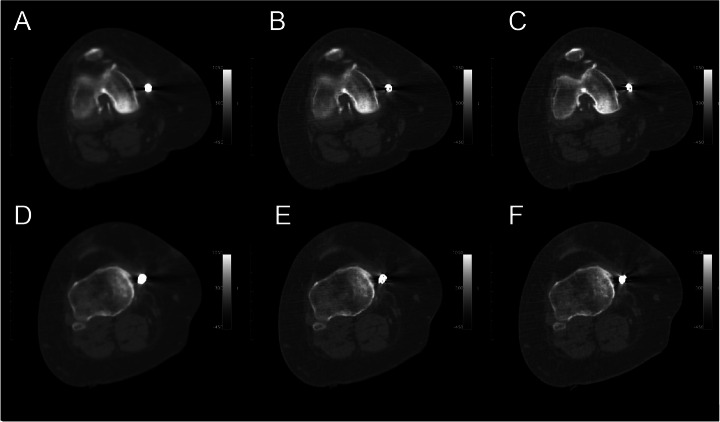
Low-dose CT scan of a female patient (48 year) with an Atlas® Knee System in the right joint. Multiple reconstructions from the same CT detector raw data (120 kV, 55 mA, *t*_rot_ = 0.8 s, pitch = 1.375). Reconstruction is shown for the region of femur (a‑c) and the tibia (d‑f). CT reconstruction was performed with setup a, d “S Plus” (slicing = 3.75 mm), b, e “B+ Plus” (slicing = 3.75 mm), and c, e “Bone Plus IQE” (slicing 1.25 mm)
